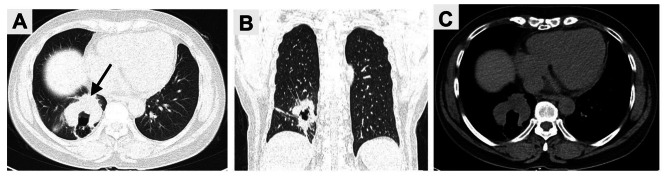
Follow-up CT examination on May 11, 2022 showed that the mass was obviously enlarged and the solid part increased, and its size was about 51 mm×61 mm (black arrow). (A) Axial lung window; (B) Coronal lung window; (C) Axial soft tissue window
